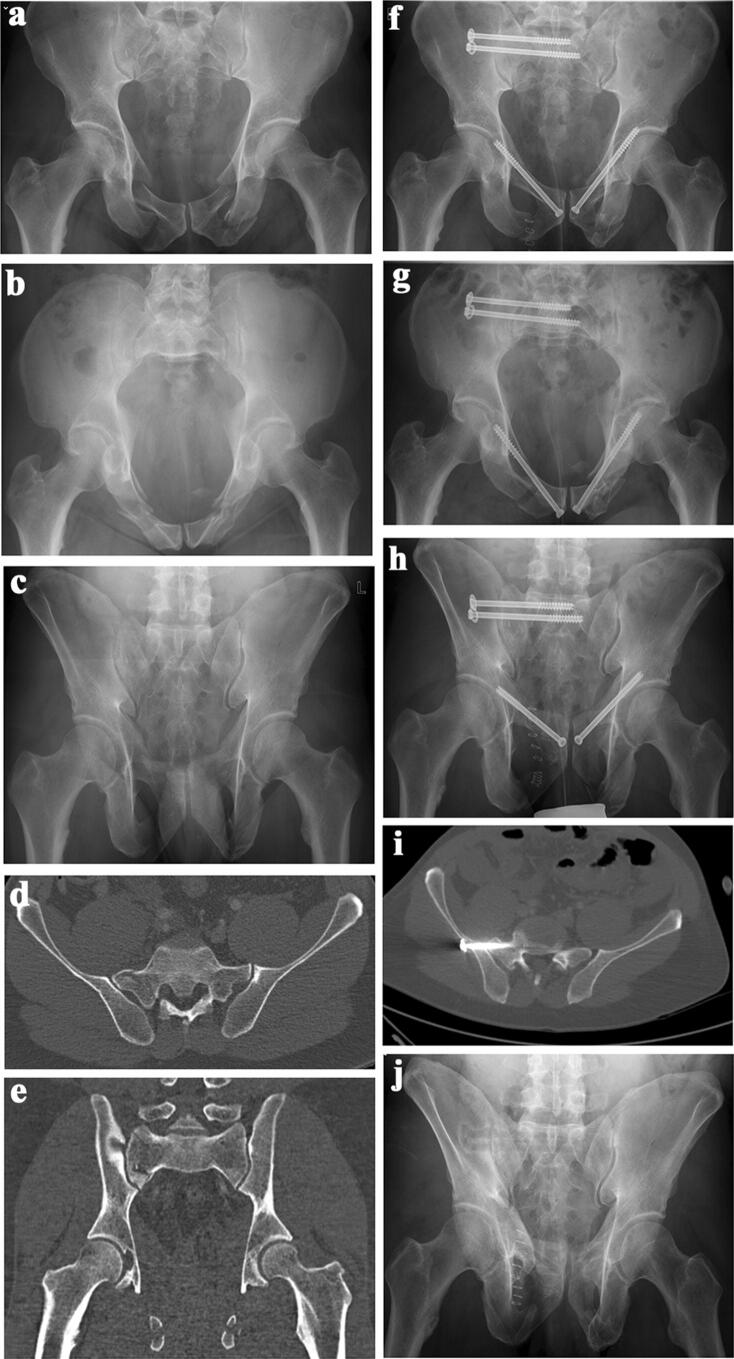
a 52-year-old motorcycle driver was hit by a car. AP-pelvic overview showing bilateral superior and inferior pubic rami fracture. A fracture of the posterior pelvis is not visible. b Pelvic inlet view. c Pelvic outlet view. There is a right-sided sacral fracture running through the neuroforamen S1. d CT-slice through the posterior pelvis. A complete fracture of the right sacral ala is visible. The upper sacrum is dysmorphic. e Coronal CT-reconstruction showing the right-sided sacral fracture. f Postoperative AP-pelvic overview. The sacral fracture was stabilized with two iliosacral screws. The superior pubic rami fractures were stabilized with two retrograde transpubic screws. g Pelvic inlet view. h Pelvic outlet view. i CT-scan through the posterior pelvis. One screw is perforating the anterior cortex of the ilium and lateral sacrum at the iliosacral joint. j Pelvic outlet view after removal of the implants
